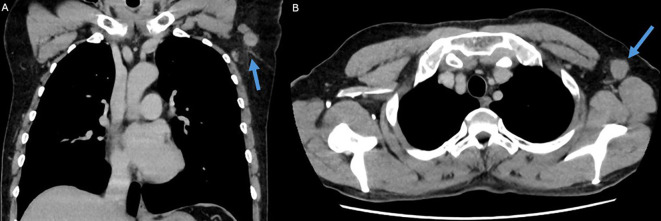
CT imaging demonstrating a tumor with adjacent nodal involvement in the left axilla, located deep to the previously excised skin nodule. (a). Coronal view (b). Axial view.
